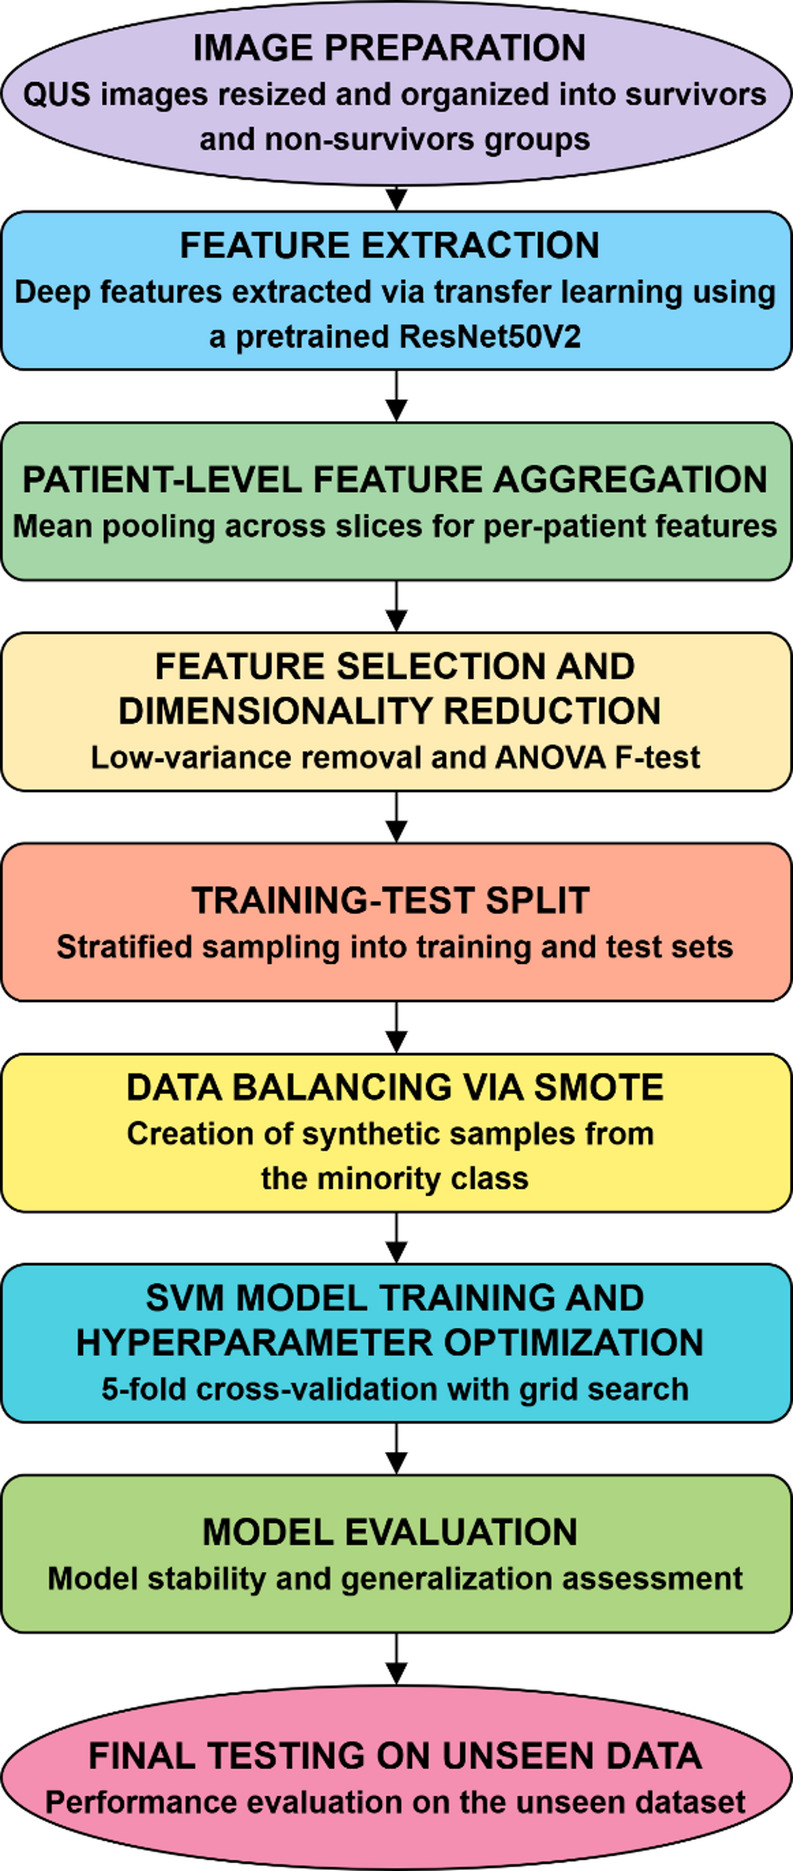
Overview of the machine learning pipeline for the Prediction of Three-Year Survival in Locally Advanced Breast Cancer Patients Using Quantitative Ultrasound Imaging. The pipeline includes resizing images and organizing them into two groups (survivors and non-survivors). This is followed by extracting deep features using a pre-trained ResNet50V2 model. The extracted features undergo patient-level aggregation followed by dimensionality reduction before being split into training and testing sets. The SMOTE technique handles class imbalance, while the SVM classifier is trained via 5-fold cross-validation with hyperparameter tuning. The model’s performance is assessed through cross-validation and an independent test set using performance metrics.
